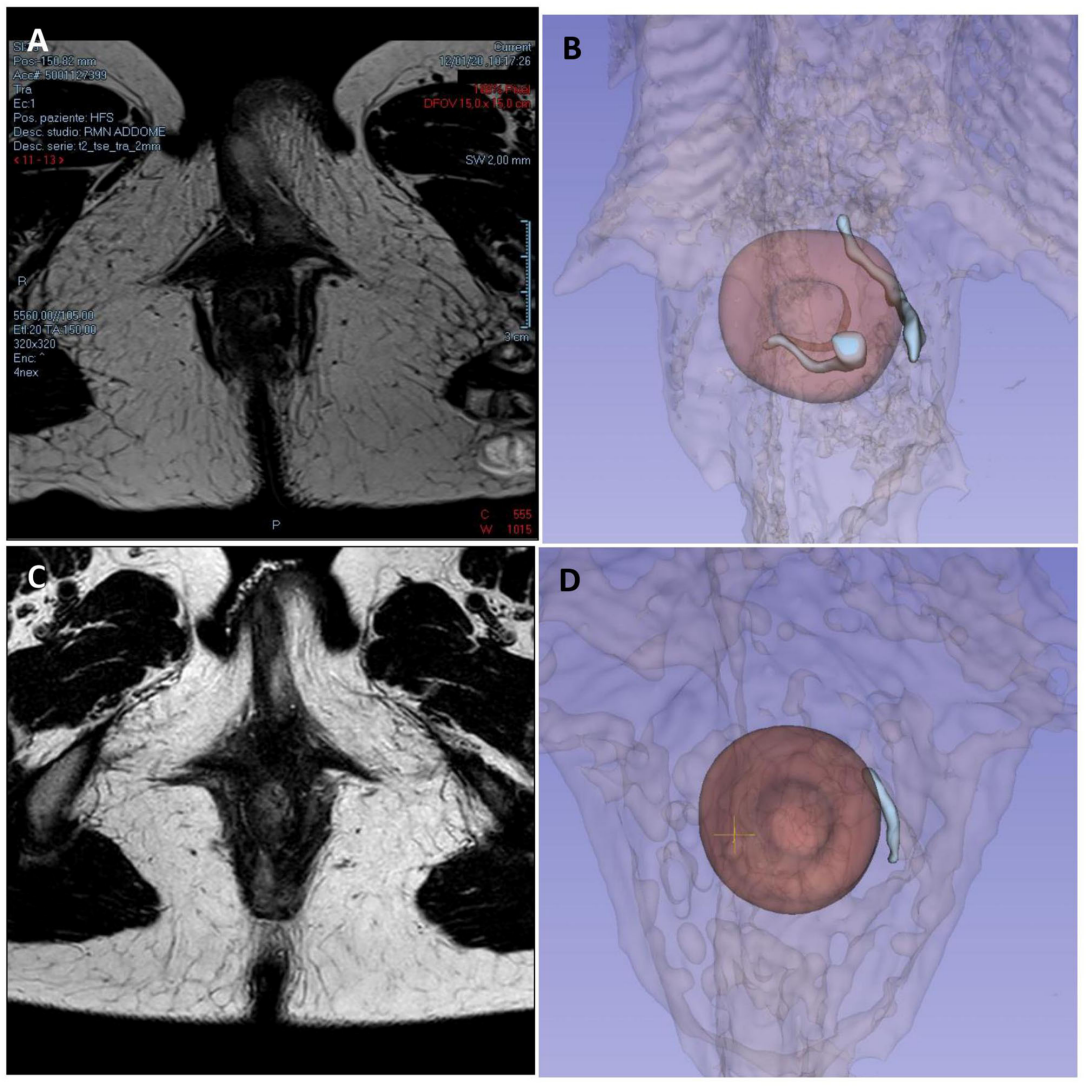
Case 3 imaging pre- and post-surgery. Panels (A, B) show preoperative pelvic magnetic resonance imaging and 3D reconstruction showing a trans-sphincteric fistula starting from the left wall of the anal canal going forward up to the homolateral part of the vulva, with diffuse subcutaneous tissue imbibition but without abscesses, and a fistula in the posterior wall of the anal canal with an inter-sphincteric course. Panels (C, D) show 6-month MRI follow-up, with the persistence of the trans-sphincteric fistula toward the vulva but without signs of inflammation or collections.
